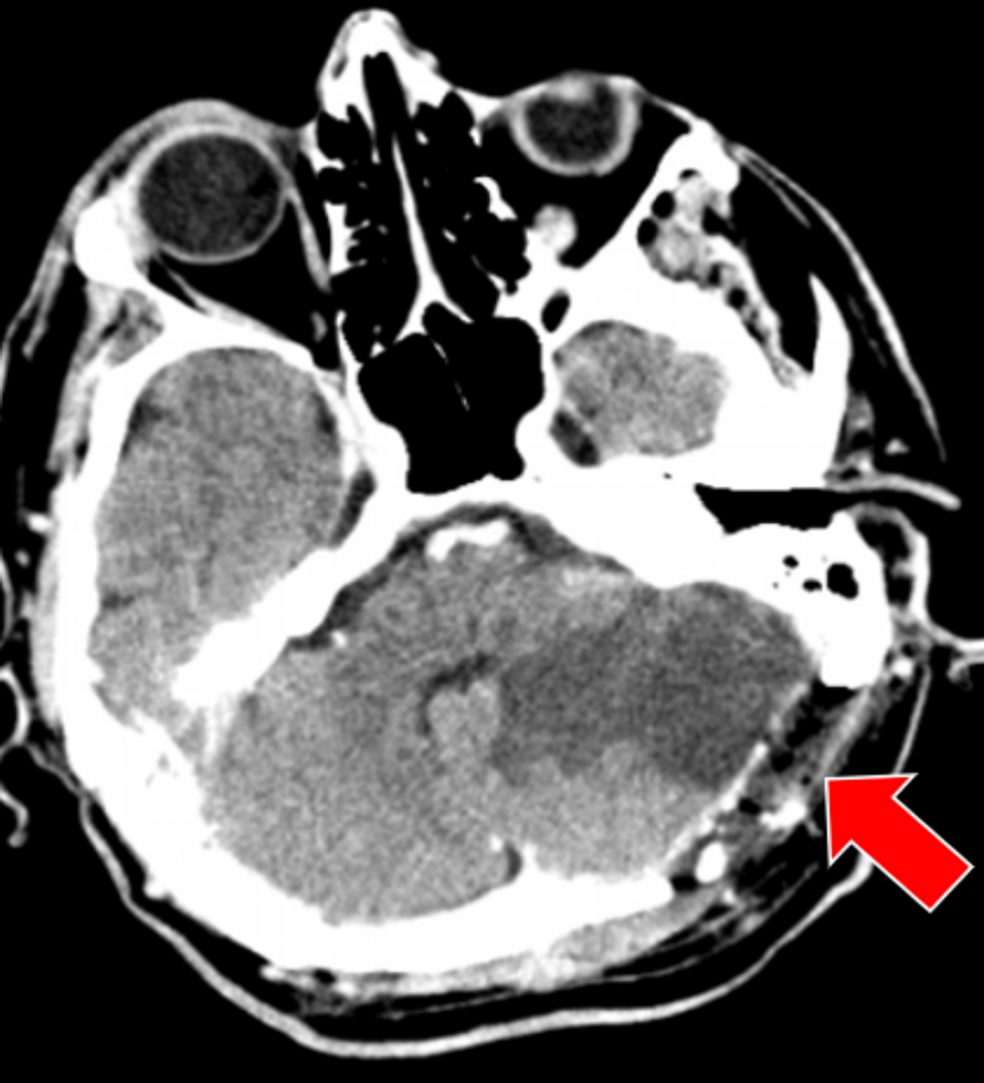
Postoperative CT scan, axial section, that confirmed the gross total resection of the tumor
